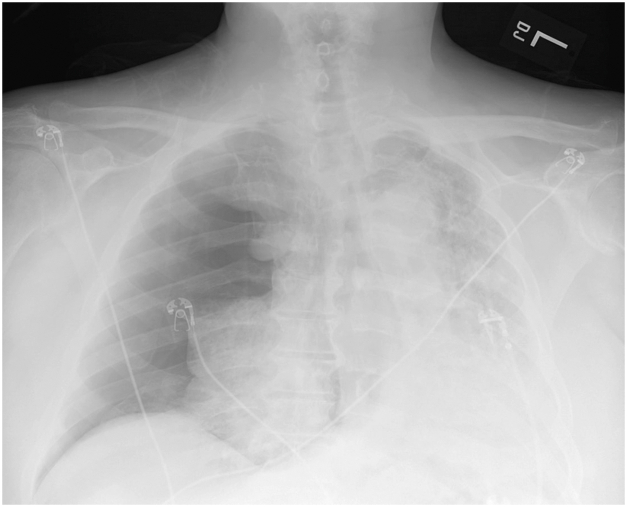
Chest radiography revealing large right pneumothorax.
